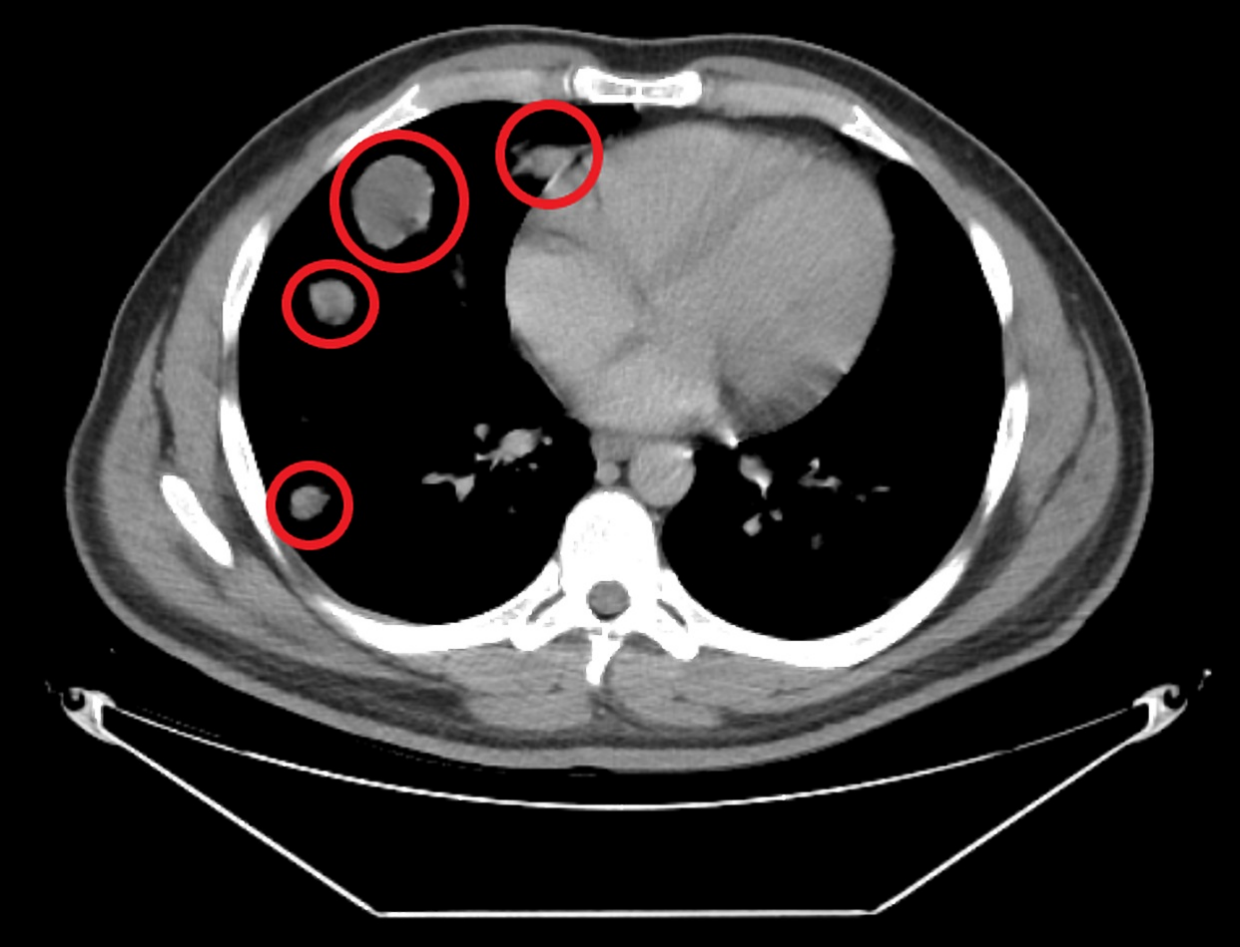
Chest computed tomography (CT) scan showing multiple metastatic pulmonary lesions (red circles) on the right side
